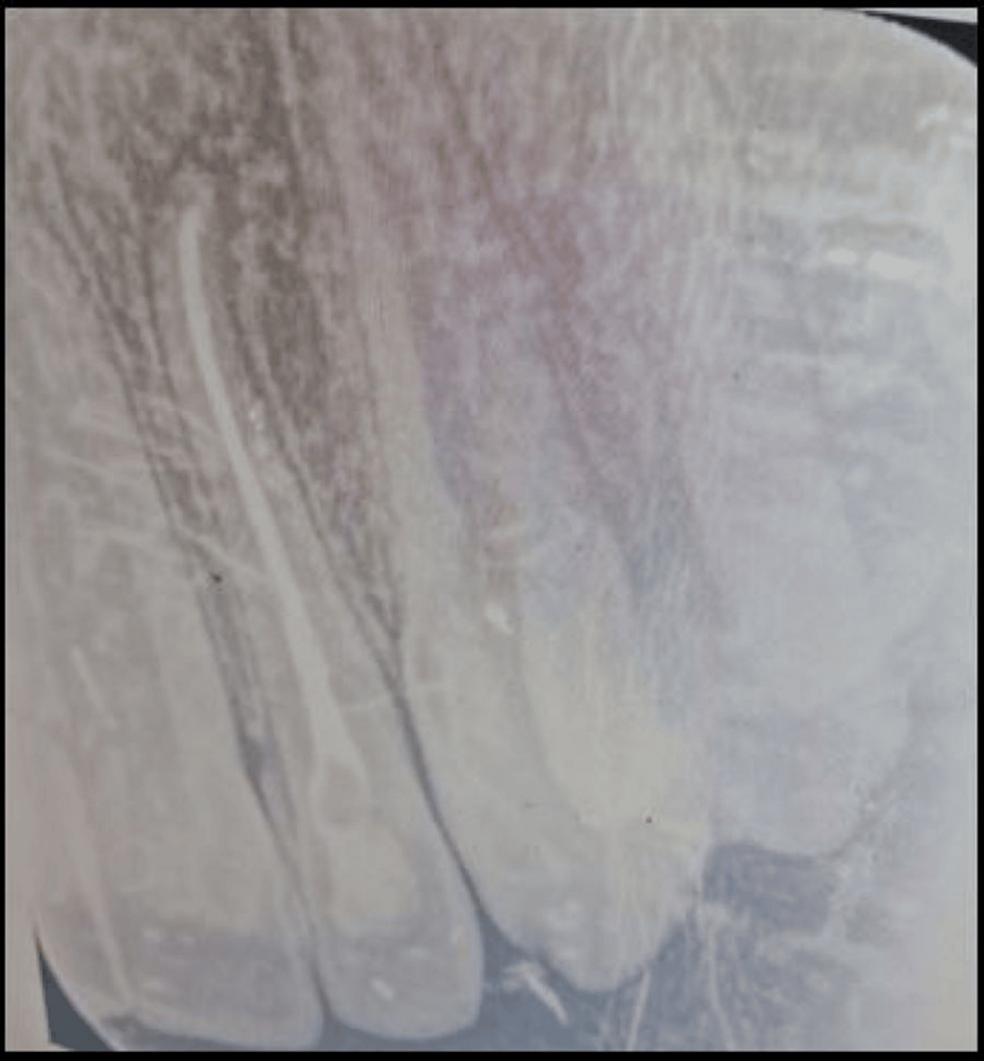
IOPAR at the six-month follow-up IOPAR showing complete healing at the periapical region of #22. IOPAR: Intraoral periapical radiograph.
